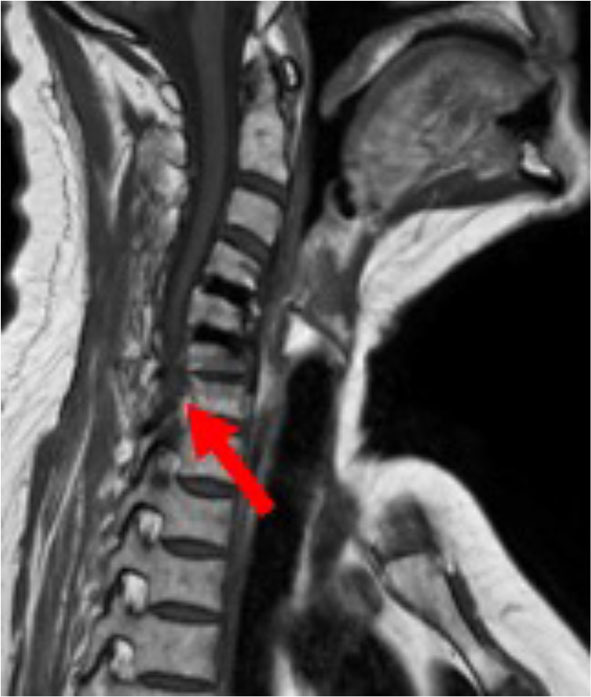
MRI double level EIT CIF cage 2 years postop Arrow indicates spinal stenosis C6/C7.
